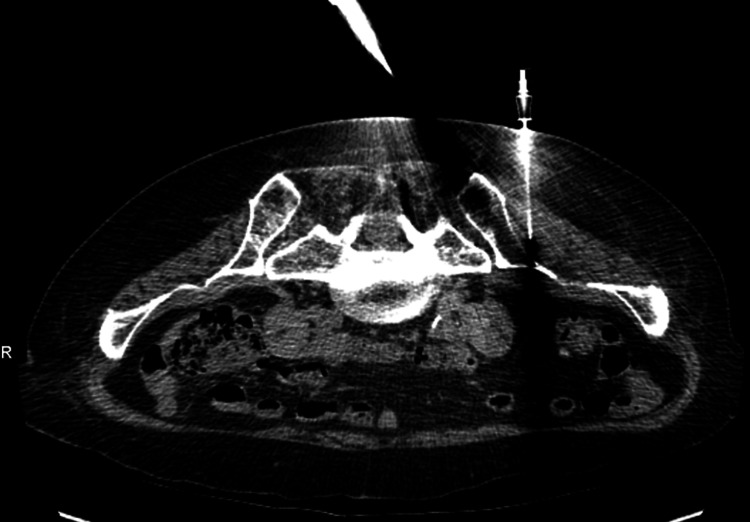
CT-guided aspiration of left iliac bone/left gluteal abscess Needle identifying site of sample extraction.
